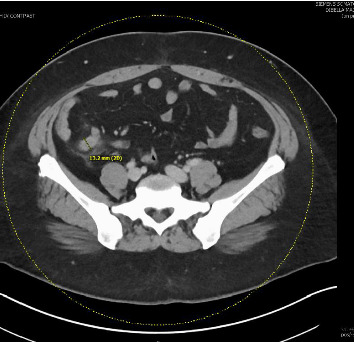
CT scan. Distention of the appendix up to 1.3 cm in caliber with periappendiceal inflammatory fat stranding, suggestive of uncomplicated acute appendicitis. No bowel obstruction.
